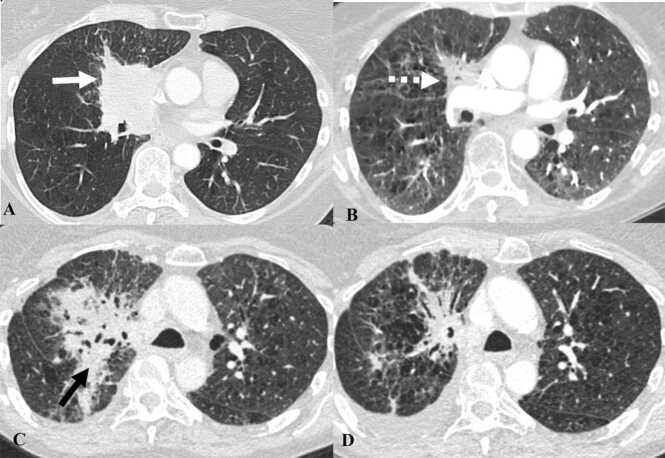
Immune-mediated radiation recall pneumonitis in a 65-year-old man with non-small cell lung cancer treated with Durvalumab therapy. Contrast-enhanced axial chest CT images demonstrate the following: A. Right perihilar mass consistent with biopsy proven non-small cell lung cancer (white arrow). B. Patient was treated with radiation and chemotherapy (cistplatin+etoposide) after which the primary tumor decreased in size (dashed white arrow). C. The patient was started on Durvalumab therapy after 2 years with worsening consolidation at the site of prior radiation in the right perihilar region (black arrow), suspicious for radiation recall pneumonitis. D. Durvalumab was stopped, and the patient was placed on steroids after which the pneumonitis decreased.
